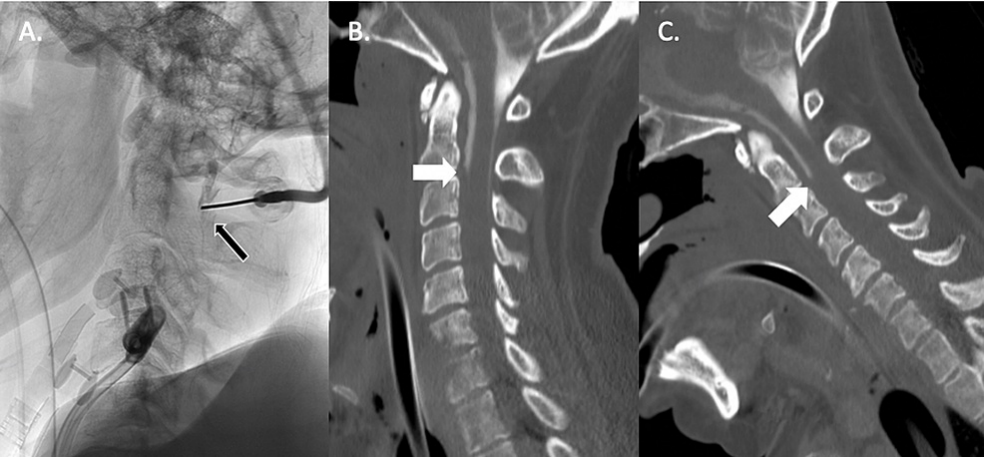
Cervical Computed Tomography Myelogram A. Lateral x-ray of the cervical spine during myelogram procedure. Percutaneous injection of iohexol 300 contrast via a 20-gauge needle at the C1-C2 level (black arrow). B. Sagittal view of computed tomography myelogram after C1-C2 lateral injection of contrast that demonstrates essentially no flow of contrast inferiorly past the level of C3. There is minimal movement down the dorsal and lateral epidural spaces at the level of C2-C3 (white arrow). Changes in discitis and osteomyelitis are again seen at C6-C7 anteriorly. C. Sagittal view with the neck in flexion to facilitate movement of contrast in the subarachnoid space. There is still no flow past the level of C3 (white arrow).
